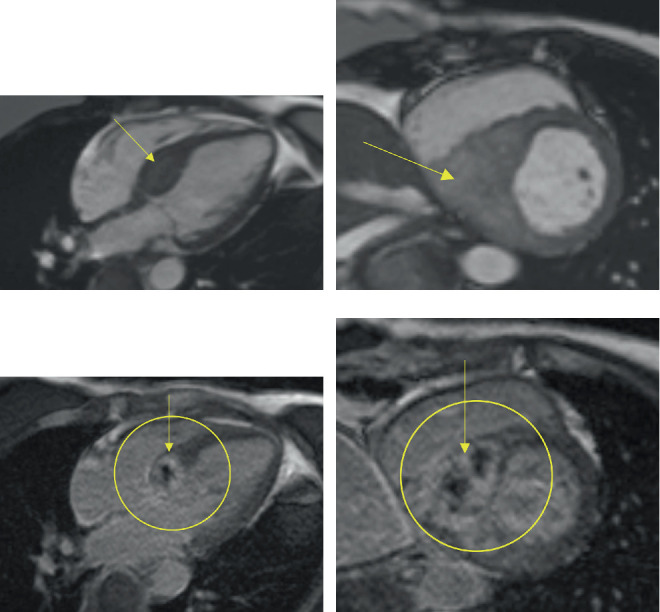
Cardiac MRI performed at an outside institution 2 years prior to presentation. (a) Four-chamber view and (b) short-axis view demonstrating a large mass within the interventricular septum. (c) Four-chamber view and (d) short-axis view postgadolinium enhancement showing central hypointensity within the mass, likely due to necrosis.
